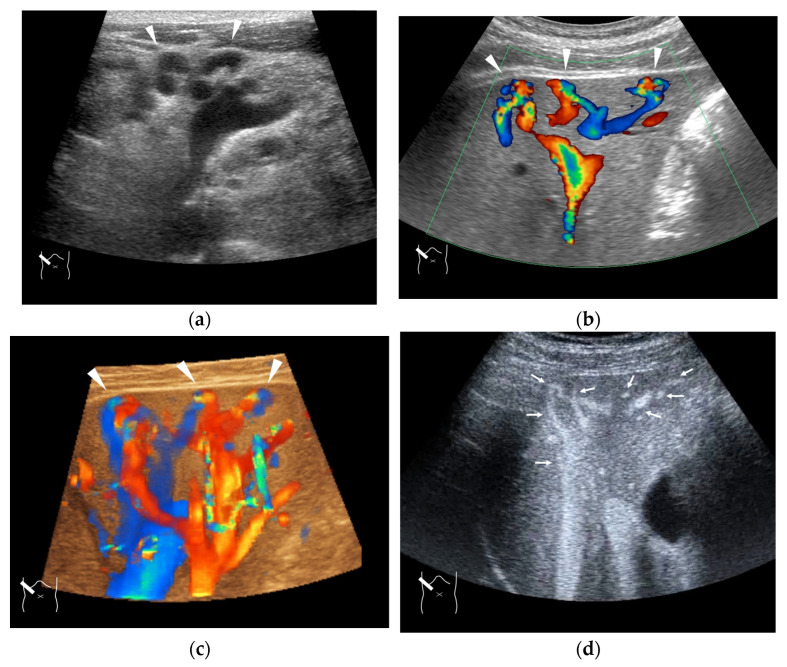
Representative case of congenital P-V shunt (type 2) in segment 6: (a) B-mode US. (b) Color Doppler US. (c) Three-dimensional color Doppler US reveals P-V shunts (arrow heads). (d) CEUS. It can be seen that all peripheral HV branches are simultaneously enhanced (arrows).
